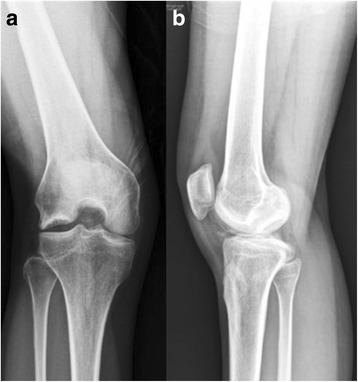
a, b Simple radiographic images of a 31-year-old female showed a large osteochondral defect on the lateral femoral condyle of right knee
